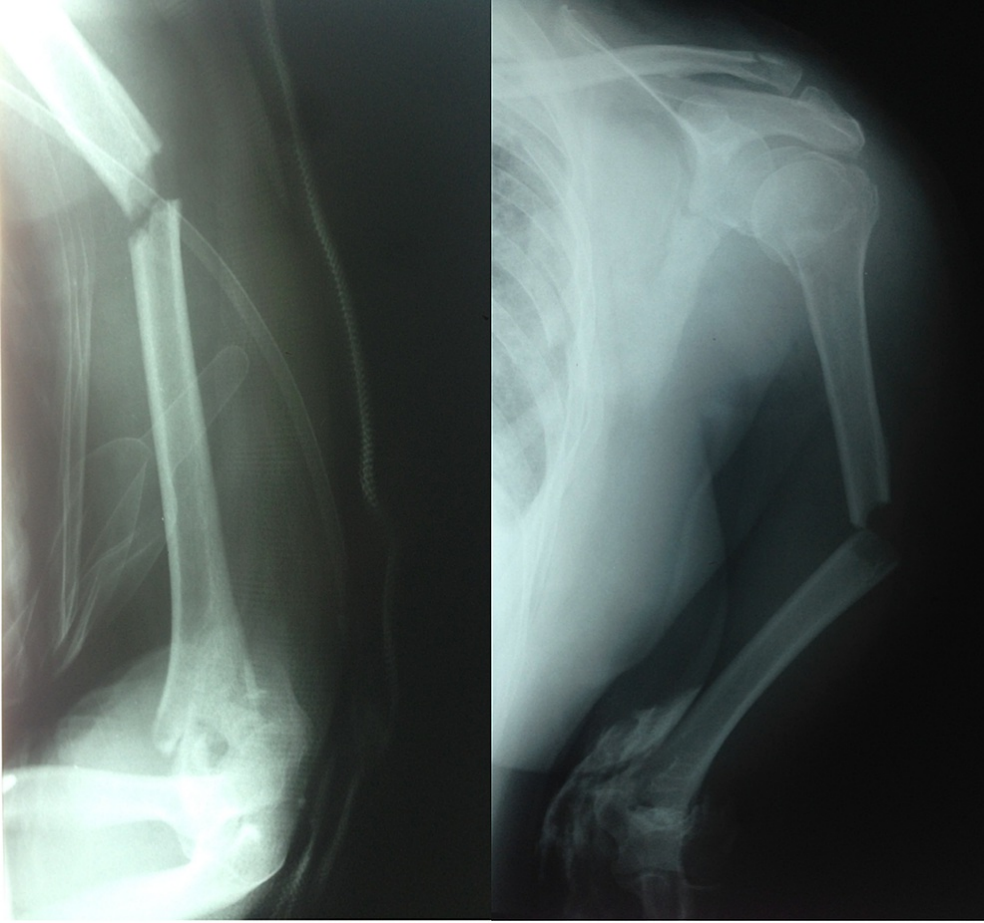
Preoperative X-rays where the proximal humerus, the middle shaft, and the supra condylar fracture are seen
